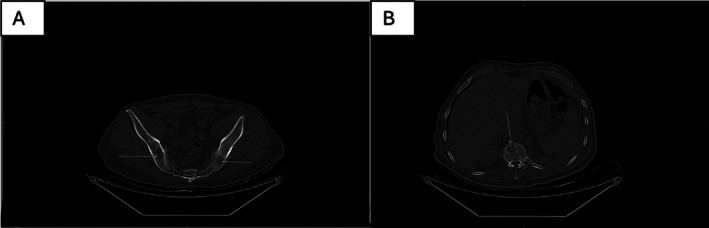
Non‐contrast axial CT images demonstrating multiple lytic lesions involving the pelvic bones and vertebral bodies: (A) bilateral iliac bone lytic lesions and (B) vertebral body lytic lesion, consistent with metastatic disease.
